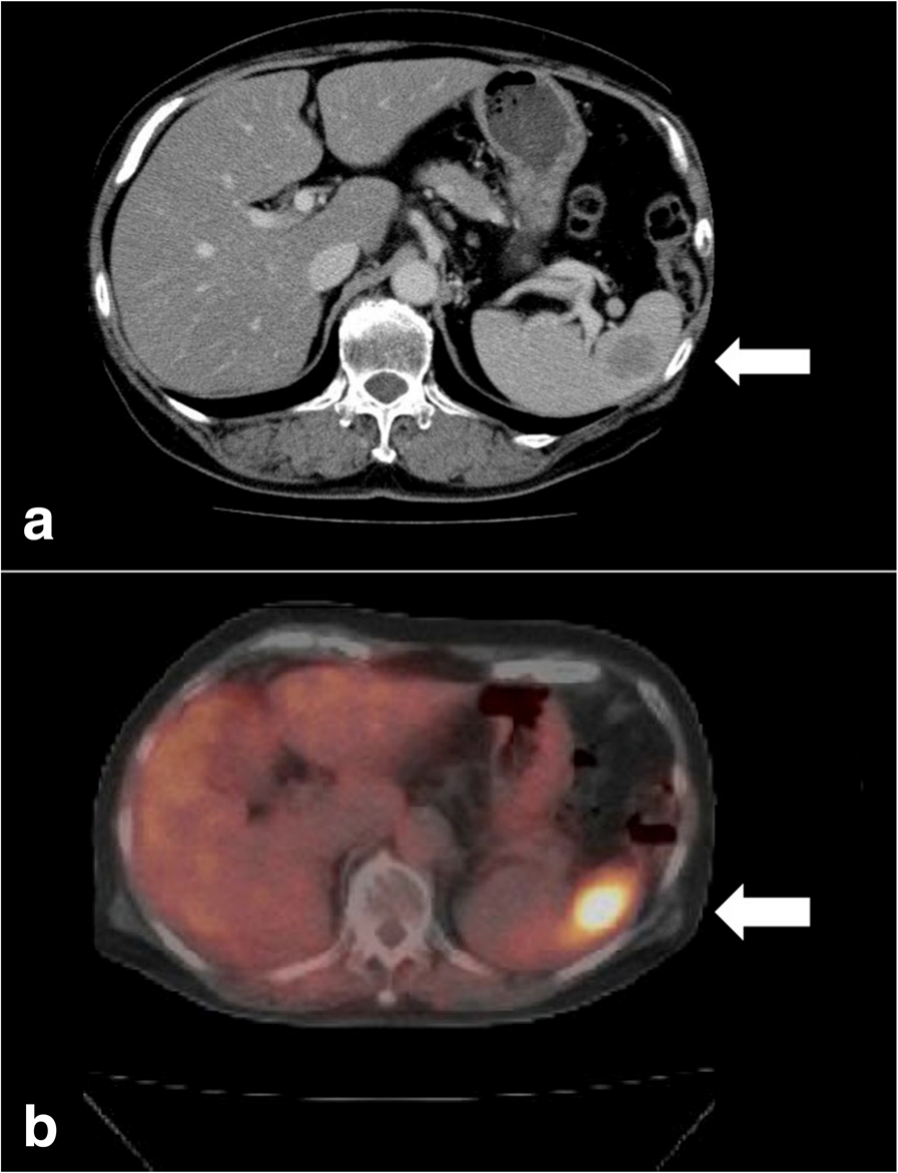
CT scan and FDG PET-CT findings. a CT scan revealed a 25-mm low-density area in the spleen (*arrow*). b FDG PET-CT revealed hypermetabolic lesions in the spleen (*arrow*)
